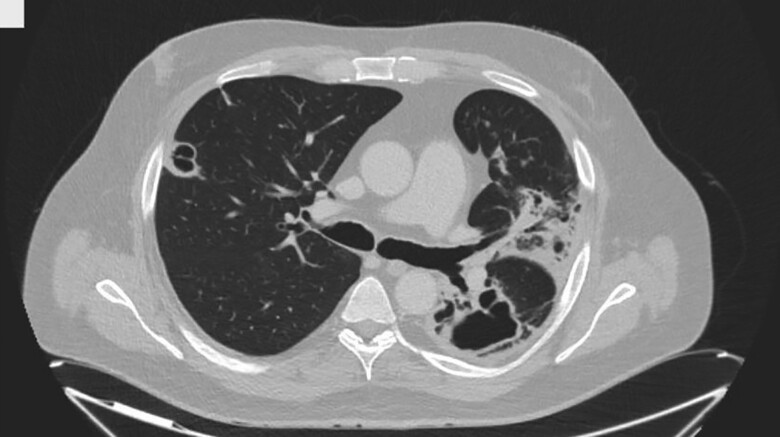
Chest computed tomography scan showing pulmonary tuberculosis with caverns connected to left main bronchus.
